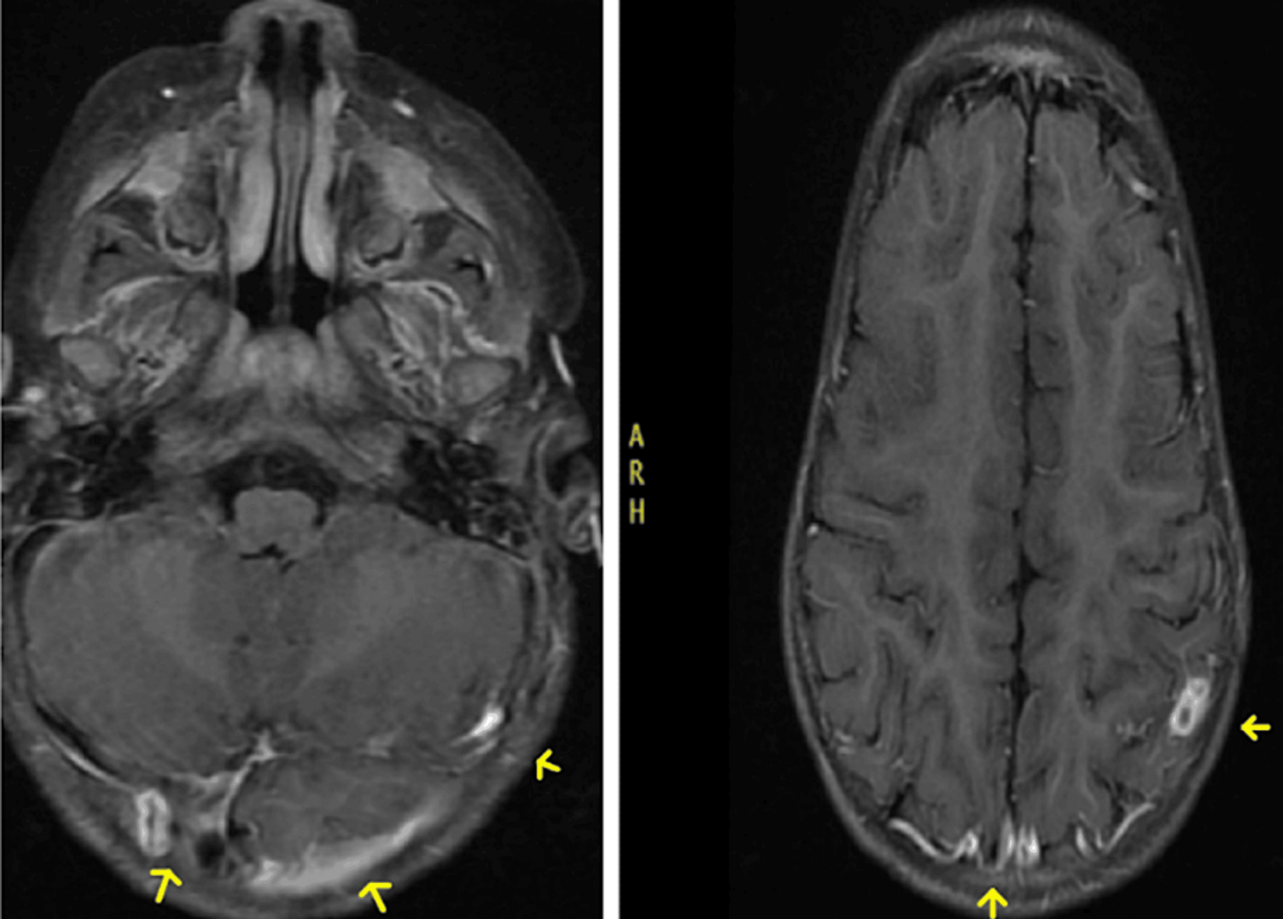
MRI showing right occipital and left posterior parietal peripherally enhancing lesions.
